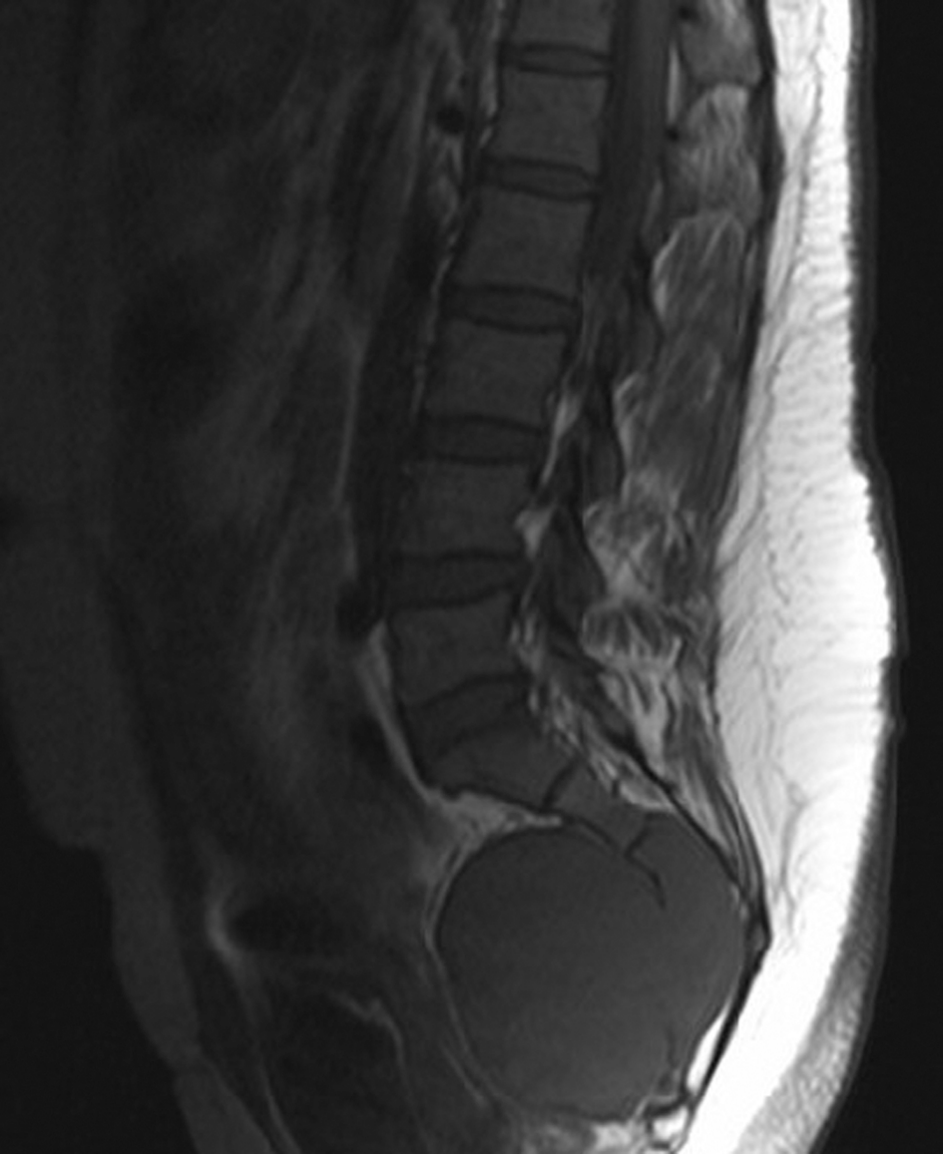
Sagittal MR T1-weighted imaging shows an expansile, well defined, homogeneously low signal mass with thinning cortex.
